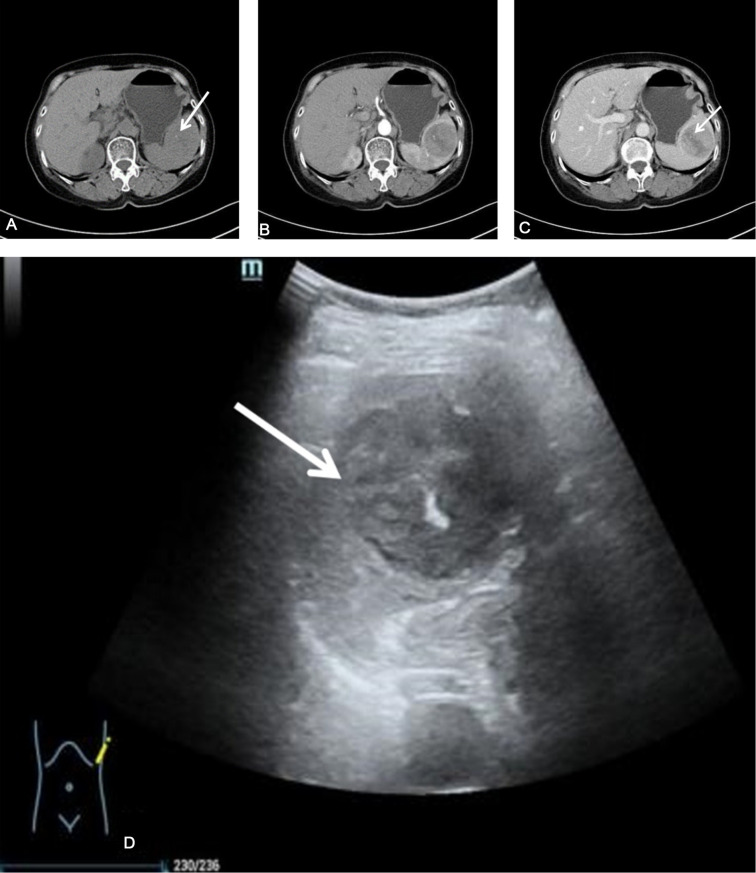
The patient's splenic CT scan. (A) Plain CT scan showed that the spleen was a round mass shadow in the parenchyma, with punctate calcifications (white arrows). The normal parenchyma was poorly demarcated. (B,C) Contrast-enhanced CT showed that the tumor enhancement was weaker than that of the normal spleen, with clear borders, and a strip-like cleft-like non-enhanced area (white arrow). (D) B-ultrasound showed a heterogeneous low echo in the spleen, with a spot-like strong echo (white arrow). CT, Computed Tomography.
